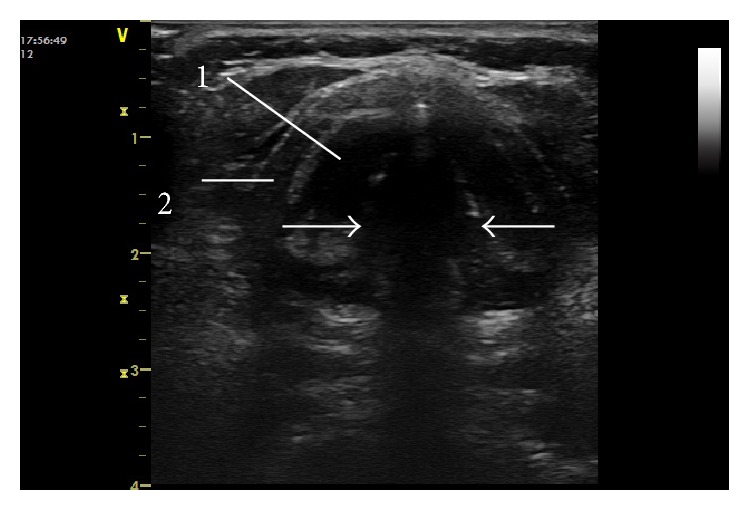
Ultrasound imaging of the relaxed vocal cords (white line with arrows). (1) Vocal muscle. (2) Thyroid cartilage.
